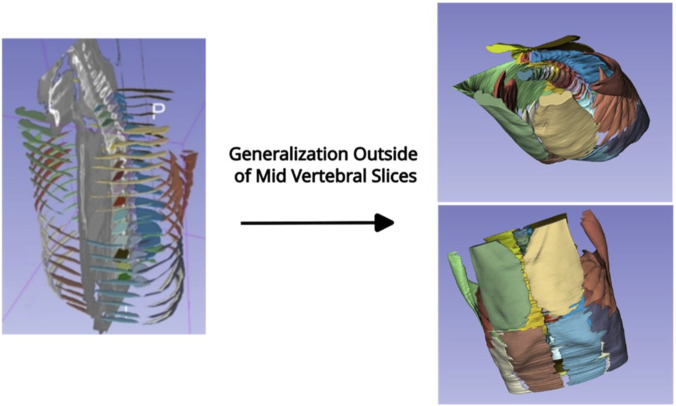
Shows the 2D segmentation per vertebral level and the resulting 3D volume when inferring every slice of the volume. Horizontal banding, visible in the abdominal region as seen in the frontal view figure (lower right), is believed to be due to breathing artifacts during the CT acquisition, as no breath-hold control was performed during the radiation planning CT scan, but may merit further investigation.
